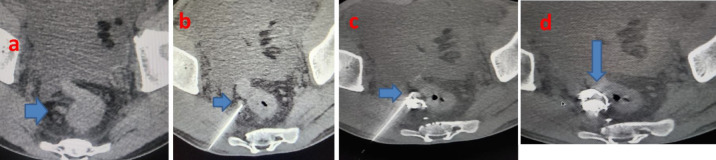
CT Guided percutaneous glue injection of rectal varix: axial nect pelvis. The glue was injected using a 22G spinal needle
